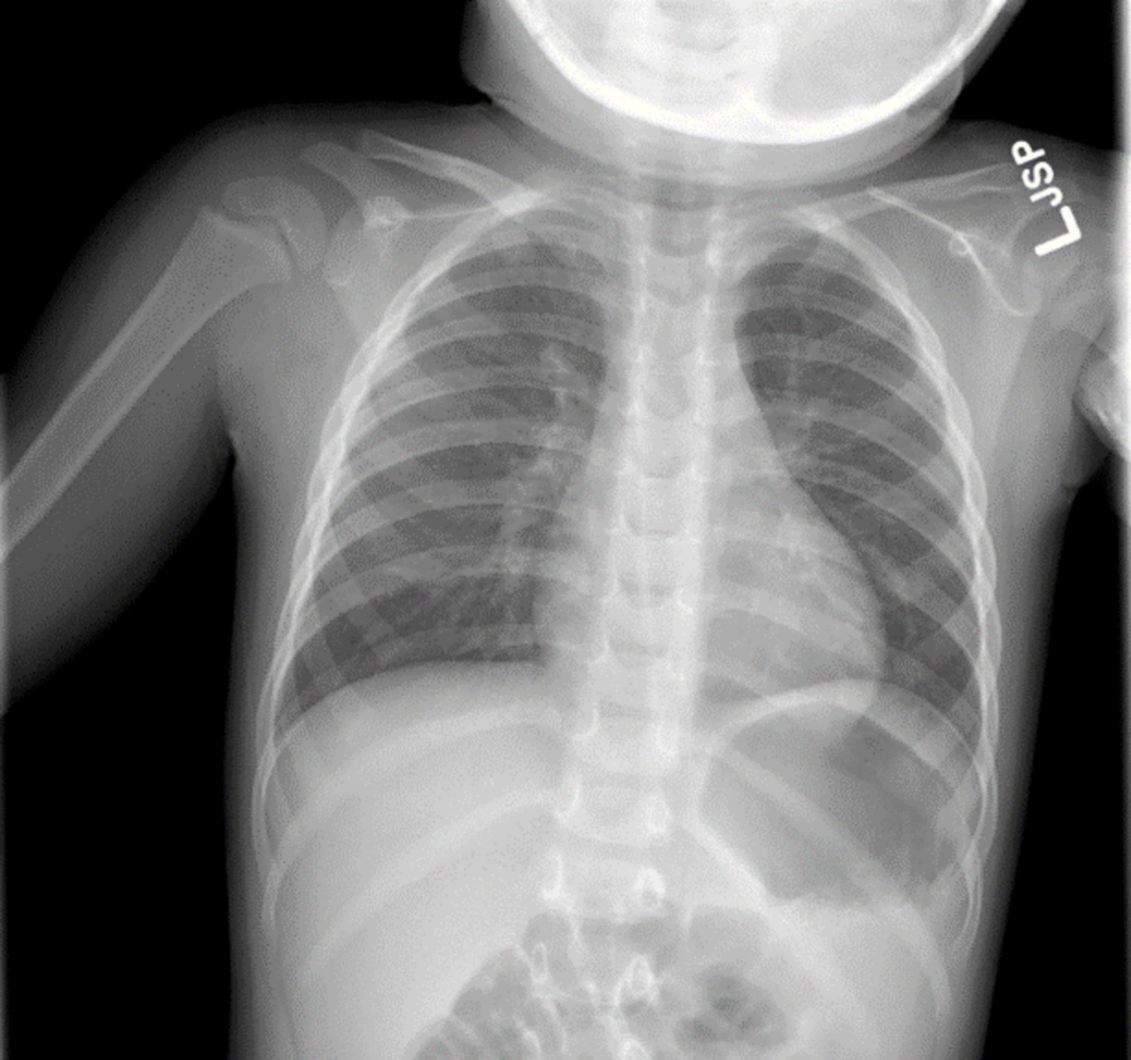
Chest radiograph in the posteroanterior (PA) view This posteroanterior chest radiograph shows no evidence of any pulmonary infiltrate or foreign body.
